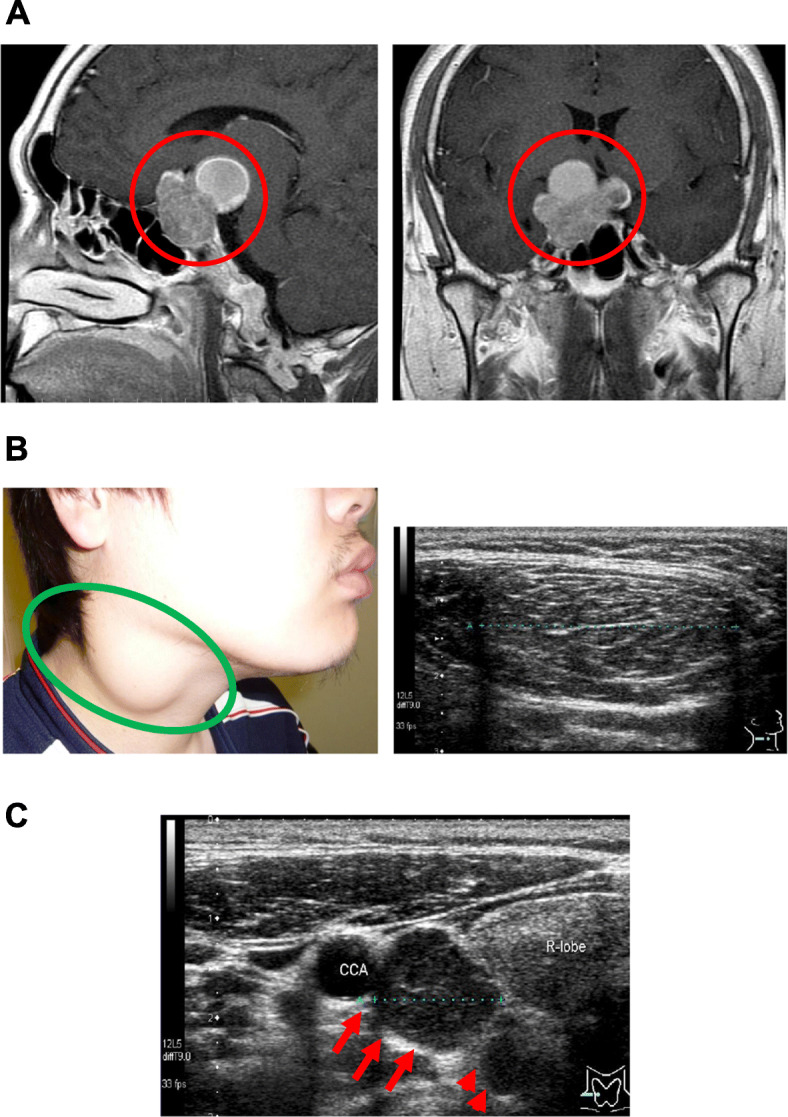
(A) Contrast-enhanced brain MRI. Neoplastic lesion was observed in the pituitary fossa (red circle). Infiltration of the tumor to all directions was observed. Its maximal diameter was approximately 50 mm, and it was a mixture of solid and cystic lesion. The cystic lesion seemed to be hematoma. Invasion of the cavernous sinus and compression of the optic nerve were also observed. (B) (Left panel) A large elastic mass was observed in right cervical region (green circle). (Right panel) The mass was clearly observed in neck ultrasonography. The view in this ultrasound image was transverse. (C) In neck ultrasonography, two parathyroid tumors were observed which maximal diameters were 38 mm (arrows) and 14 mm (arrow heads), respectively. The view in this ultrasound image was transverse
